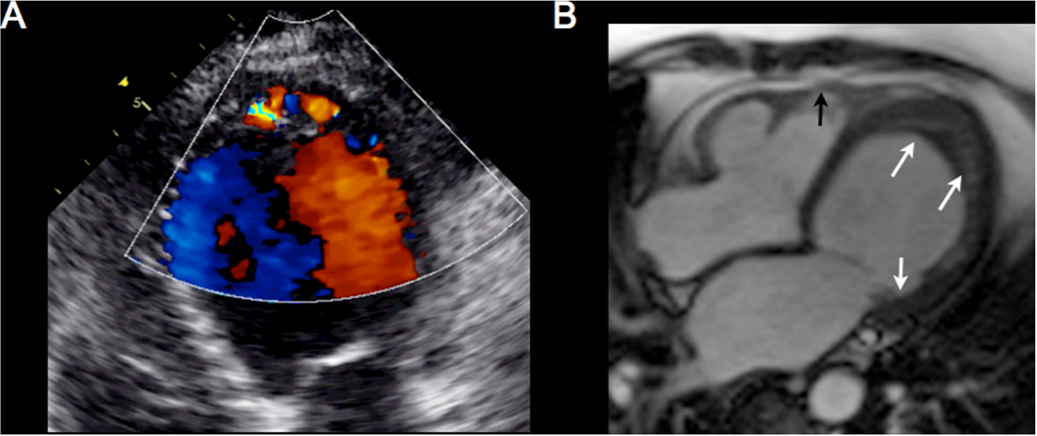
Multimodality cardiac imaging of left ventricular non compaction. A) An apical 4 chamber view on transthoracic echocardiography demonstrating trabeculations and recesses within the left ventricular apex on color Doppler consistent with left ventricular non compaction. B) A horizontal long axis steady-state free precession view on cardiac magnetic resonance imaging confirming significant hypertrabeculations involving the left ventricualr apex, lateral wall, and extension into the posterior mitral valve leaflet (white arrows). There is also evidence of dyskinesis of the right ventricular free wall that is mildly aneurysmal (black arrows).
